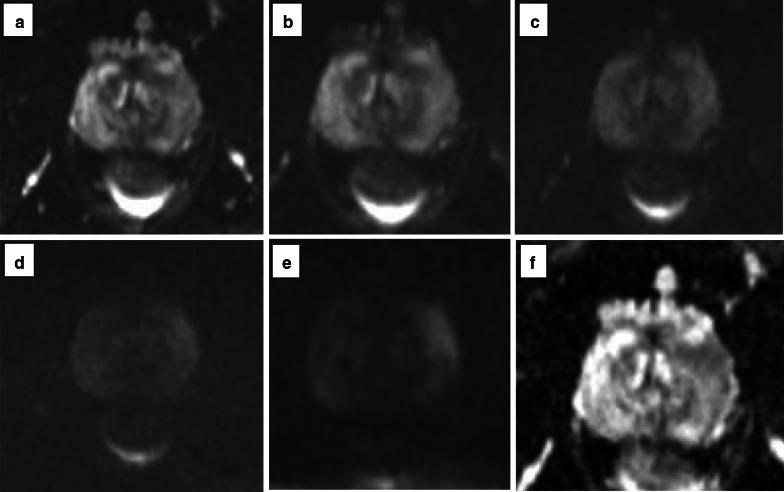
DWI at different *b* values (0–150–500–1000 s/mm^2^) in a–d, respectively. Dedicated high *b* sequence (*b* = 1400 s/mm^2^) and corresponding ADC map on a 1.5 T MR system in e and f, respectively
